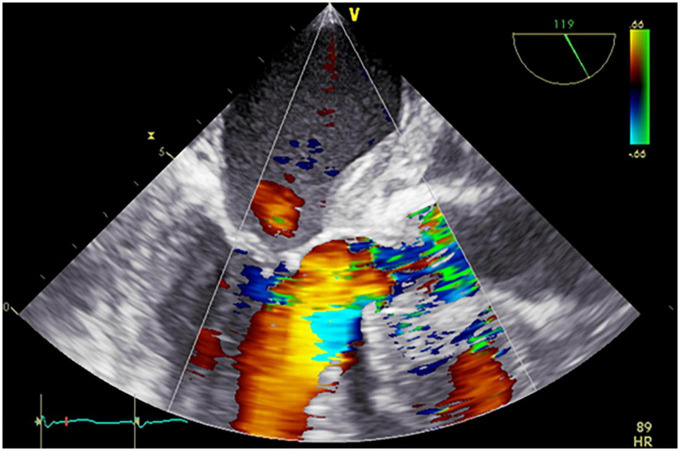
Pre-operative transesophageal echocardiogram. Severe aortic regurgitation and thickened aortic wall.
